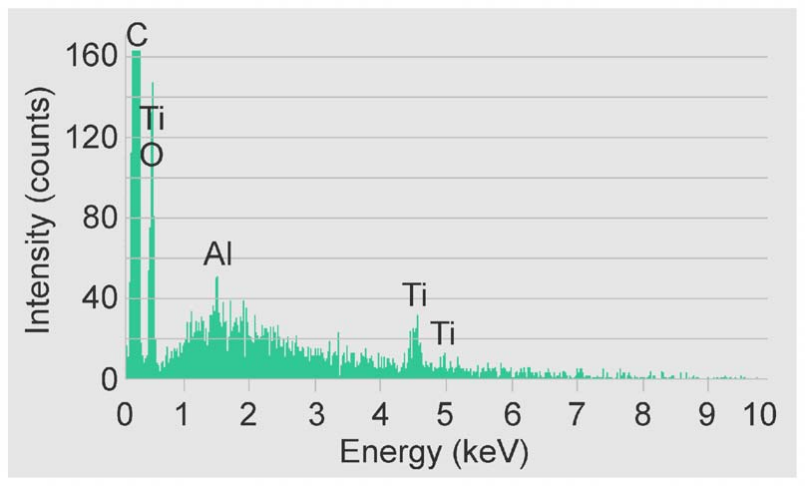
Energy Dispersive X-ray analysis. EDX confirms presence of titanium, oxygen and aluminum on the scratched-off nanotubes from surface of an anodized sample placed on carbon tape.
